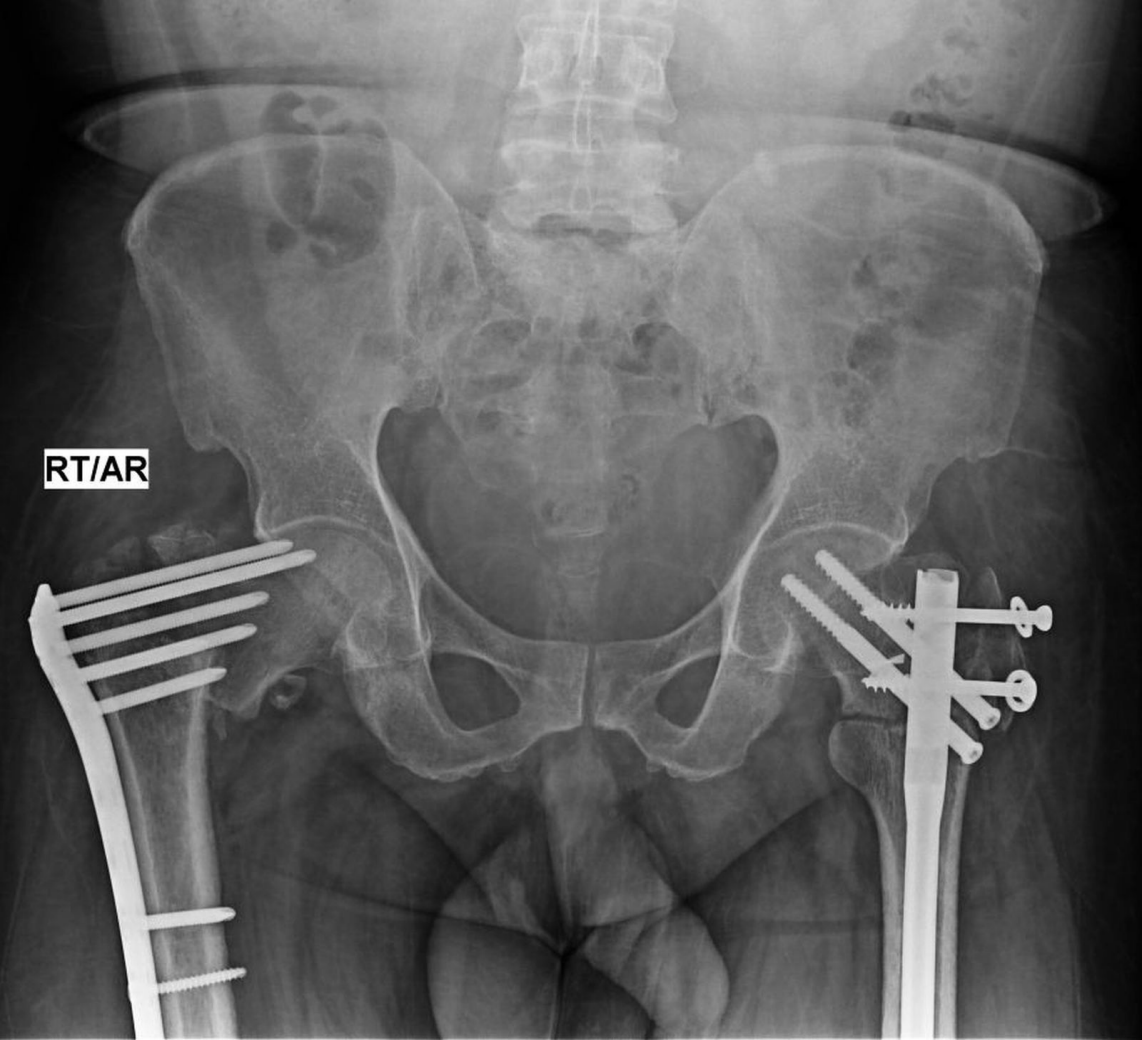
Nine months post-operative x-ray of the pelvis showing implant failure of the right hip
